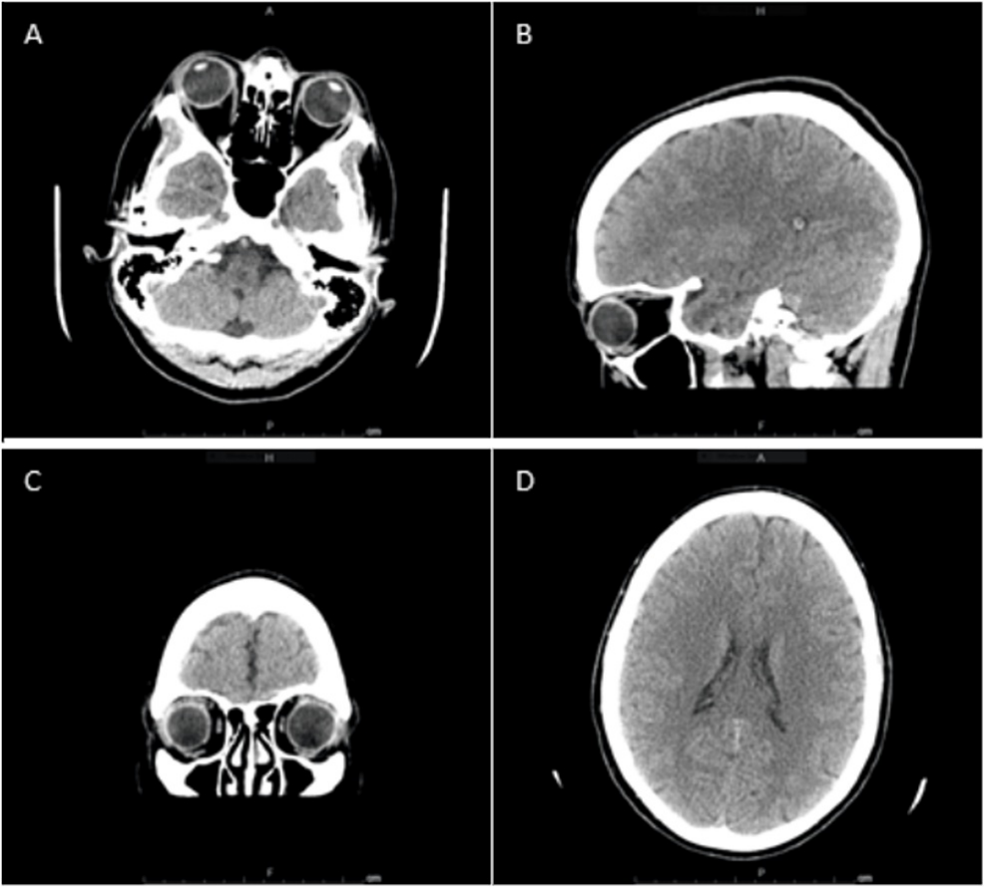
CT imaging of the head CT of the head showed no evidence of hemorrhage, no mass effect, normal attenuation characteristics of the brain parenchyma, and ventricles normal for age. (A) Transverse CT imaging showing the bilateral orbits. (B) Sagittal CT imaging showing the right orbit. (C) Coronal CT imaging showing the bilateral orbits. (D) Transverse CT imaging showing the normal ventricles CT: computed tomography
